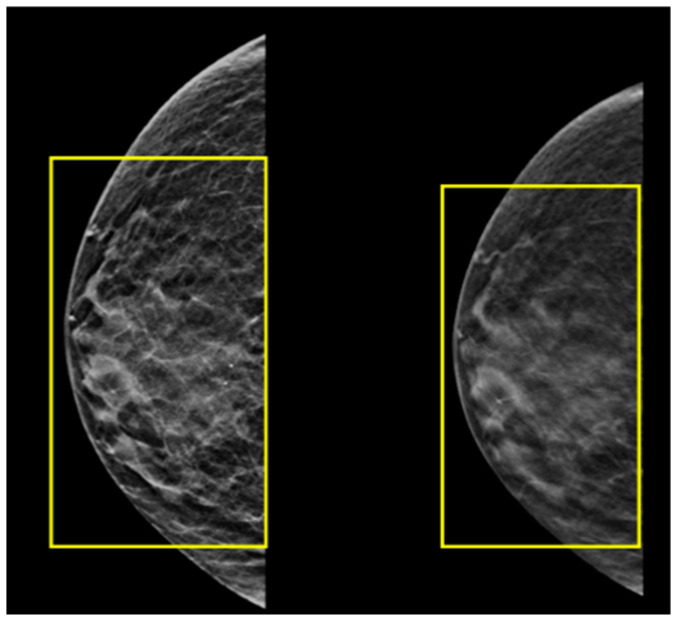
Images from the patient’s ultrasound and mammography screening of the left breast showing prominent breast tissue throughout (yellow squares).
